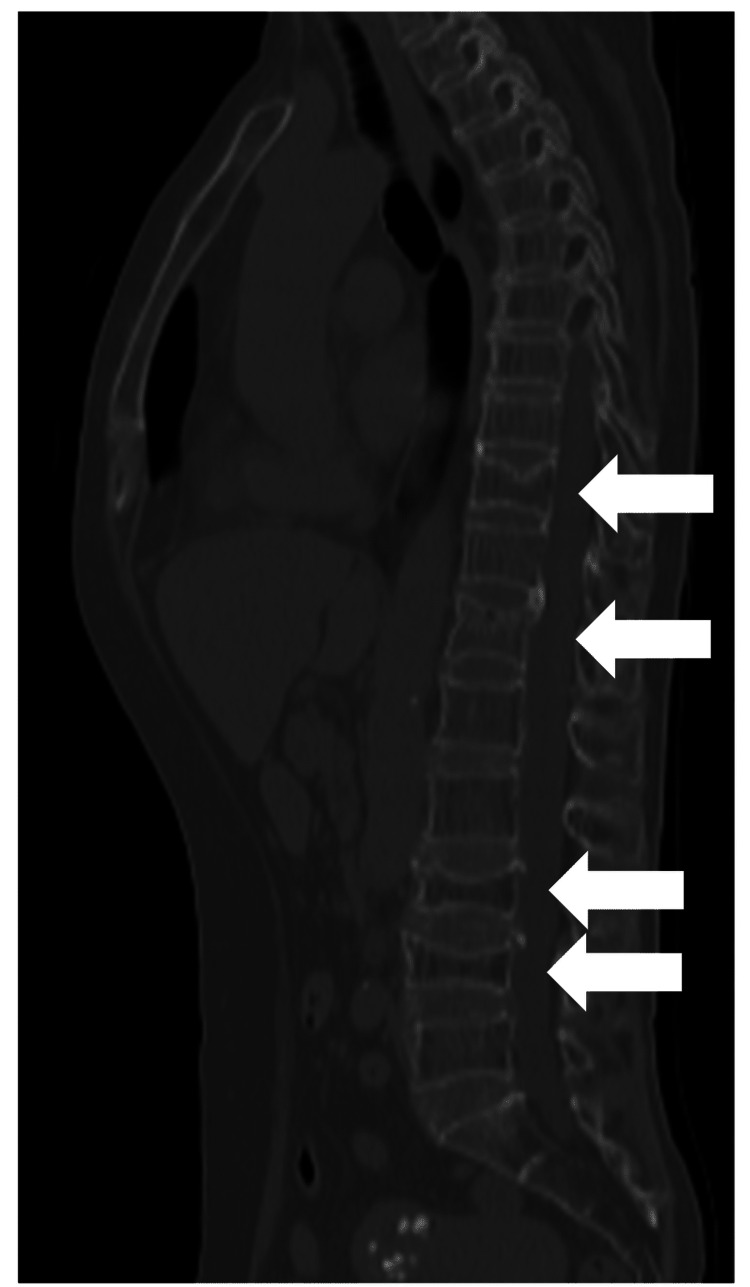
Spinal CT image A compression fracture can be observed in Th10, Th12, L3, and L4 (white arrows).
